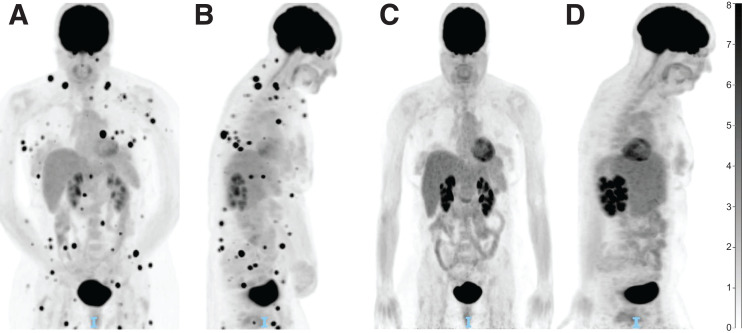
PET/CT for immunotherapy treatment response assessment. (A) PET/CT displaying innumerable intramuscular and subcutaneous soft-tissue nodules consistent with metastatic melanoma (coronal view). (B) Initial PET/CT (midsagittal view). (C) Repeat PET/CT less than 3 mo later and after 3 cycles of ipilimumab-nivolumab, showing complete resolution of soft-tissue nodules (coronal view). (D) Repeat PET/CT displaying treatment response (midsagittal view). Unit of measure for intensity bar is SUV = C(T)/[injection dose (MBq)/patient weight (kg)]).
